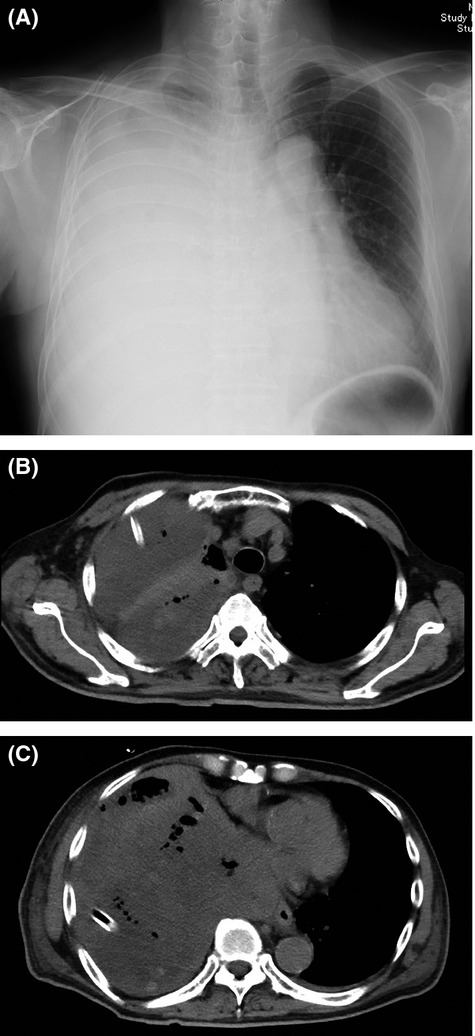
Chest radiography (A) at initial visit showed a massive pleural effusion on the right thorax. A Loculated pleural effusion was confirmed on the upper right thorax. Chest computed tomography (B, C) performed 2 weeks after admission confirmed massive enlarging loculated effusions. Two chest drainage tubes were separately inserted into the upper and lower right thorax.
